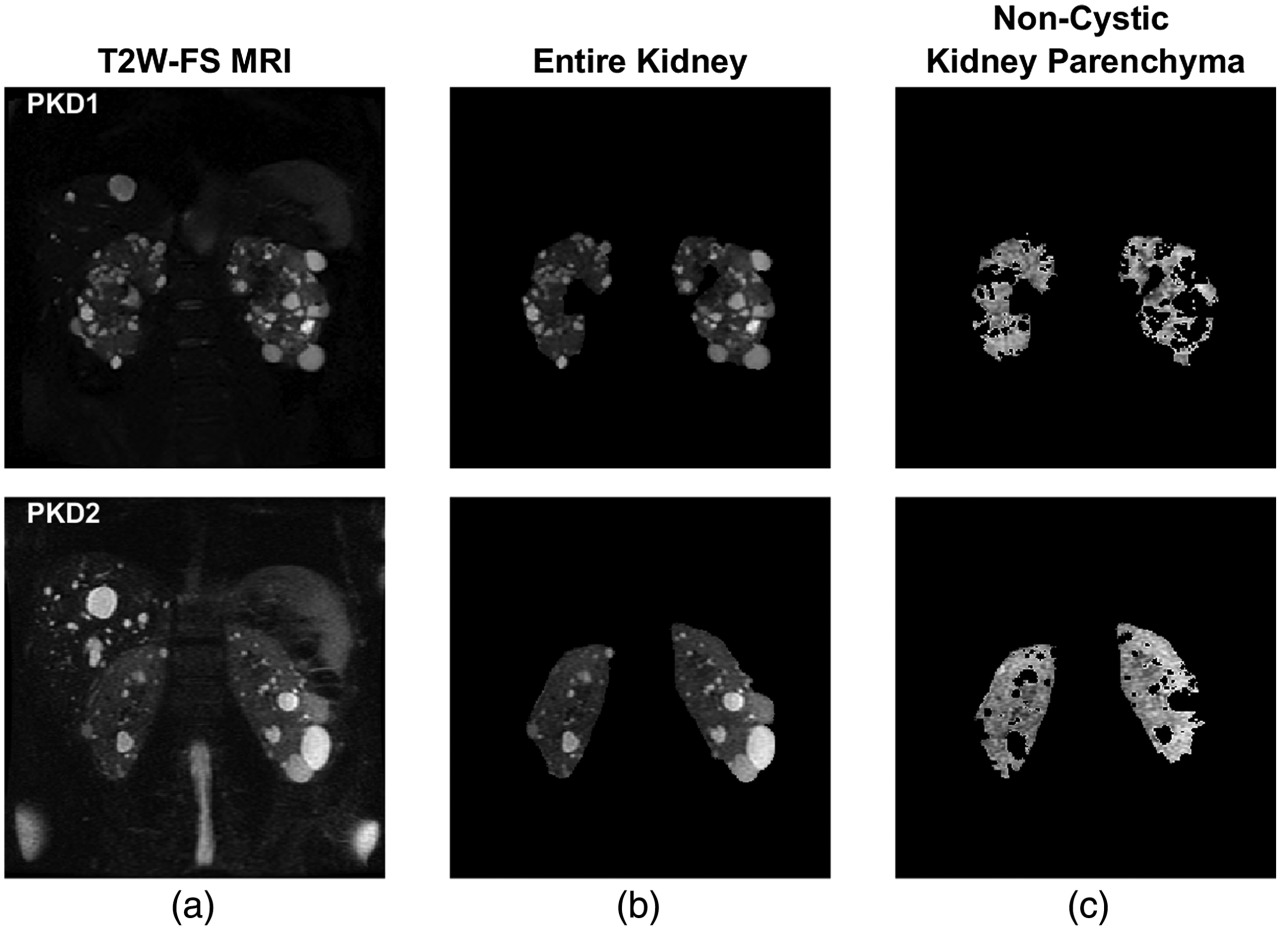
(a) Representative MRI slice, (b) the result of kidney segmentation, and (c) the result of cyst segmentation. These segmented regions were used for feature extraction for an MIC 1B PKD1 patient (top) and a PKD2 patient (bottom).
